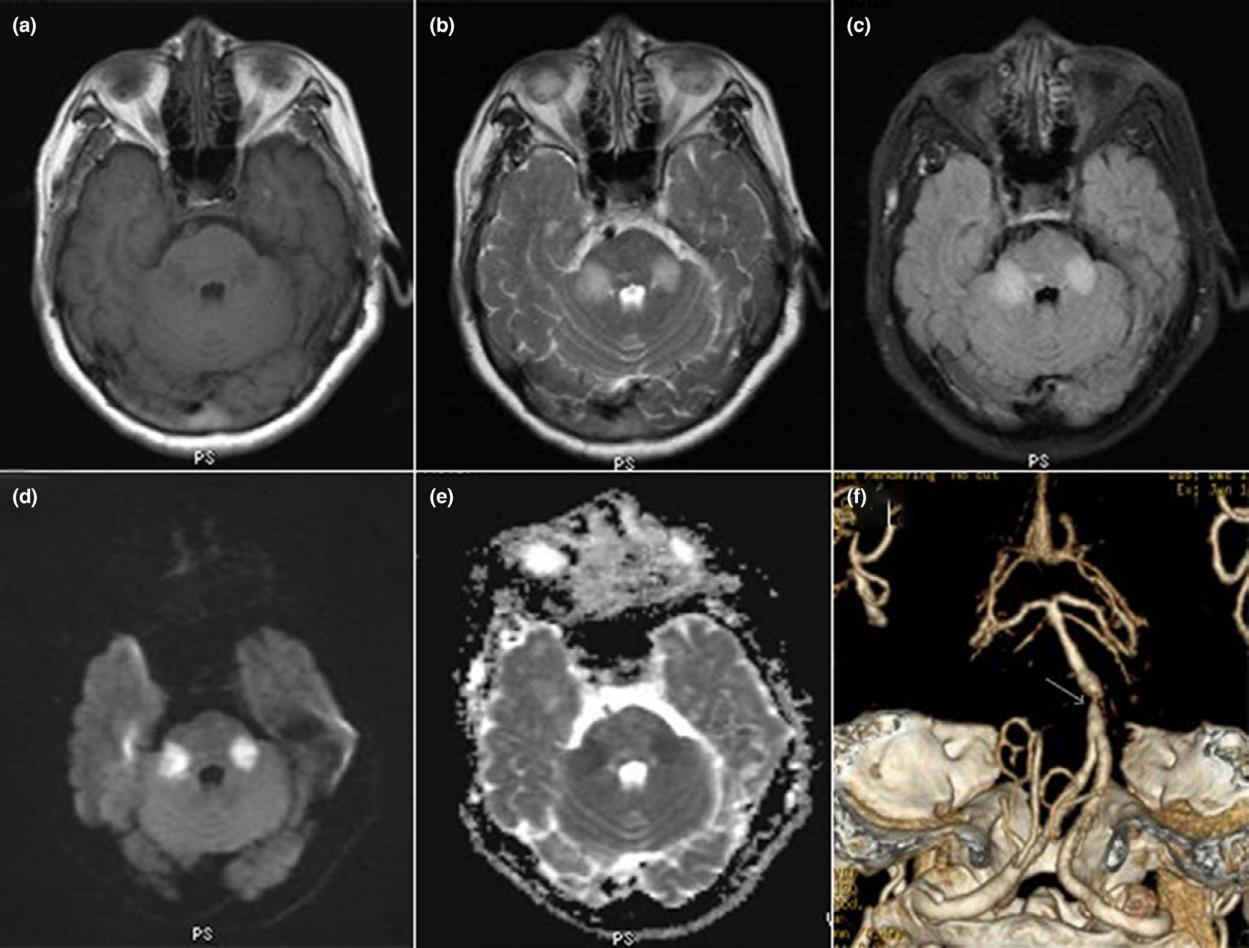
A 31‐year‐old woman with AQP4‐IgG‐seropositive neuromyelitis optica. Axial brain T1‐weighted imaging (a) shows hypointensity in both MCPs, and hyperintensity on T2‐weighted (b) and fluid‐attenuated inversion recovery imaging (c). Axial diffusion‐weighted imaging (d) demonstrates restricted diffusion signals in both MCPs, with corresponding hypointensity on the apparent diffusion coefficient map (e). Computed tomographic angiography (f) reveals severe stenosis of the basilar artery near the origin of the anterior inferior cerebellar artery (AICA; white arrow) and bilateral AICA occlusion
